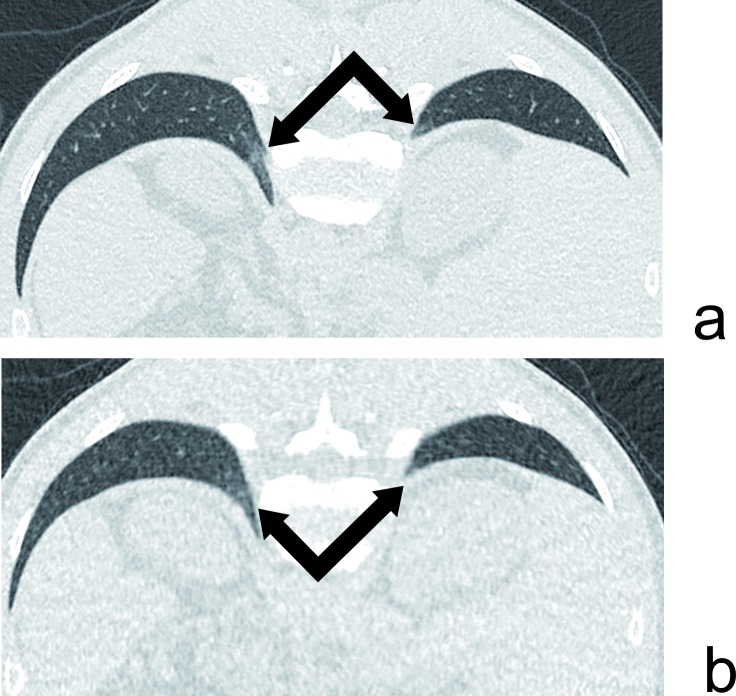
ULD CT false negative example. 47yo male with 15 years of asbestos exposure. Subtle posterior basal interstitial abnormalities related to early asbestosis are visible in the standard acquisition (a–DLP of 201mGy.cm) but indiscernible on the ULD CT (b–DLP of 19mGy.cm).
